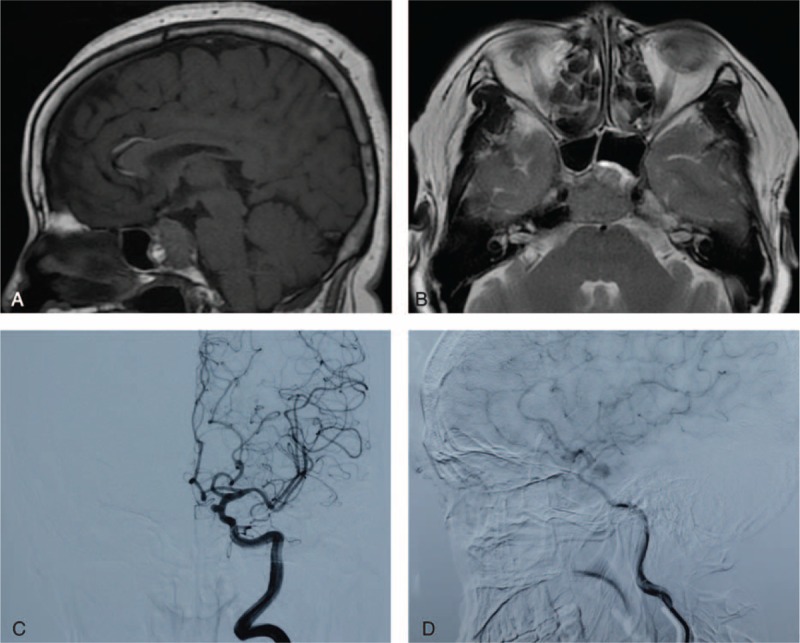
Radiological findings: sagittal T1-WI (A) and axial T2-WI (B) of MRI revealed the pituitary mass with suprasellar extension that mimicked pituitary adenoma. DSA revealed the ICA (C) and blood vessels supplying the sellar mass (D). DSA = digital subtraction angiography; ICA = internal carotid aneurysm; MRI = magnetic resonance imaging.
